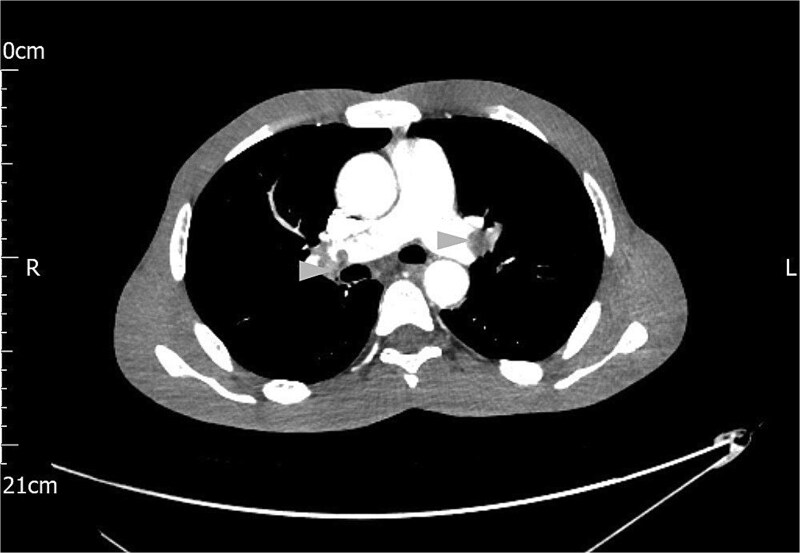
Contrast CT scan of the chest showing bilateral pulmonary artery thromboemboli (arrow heads).
